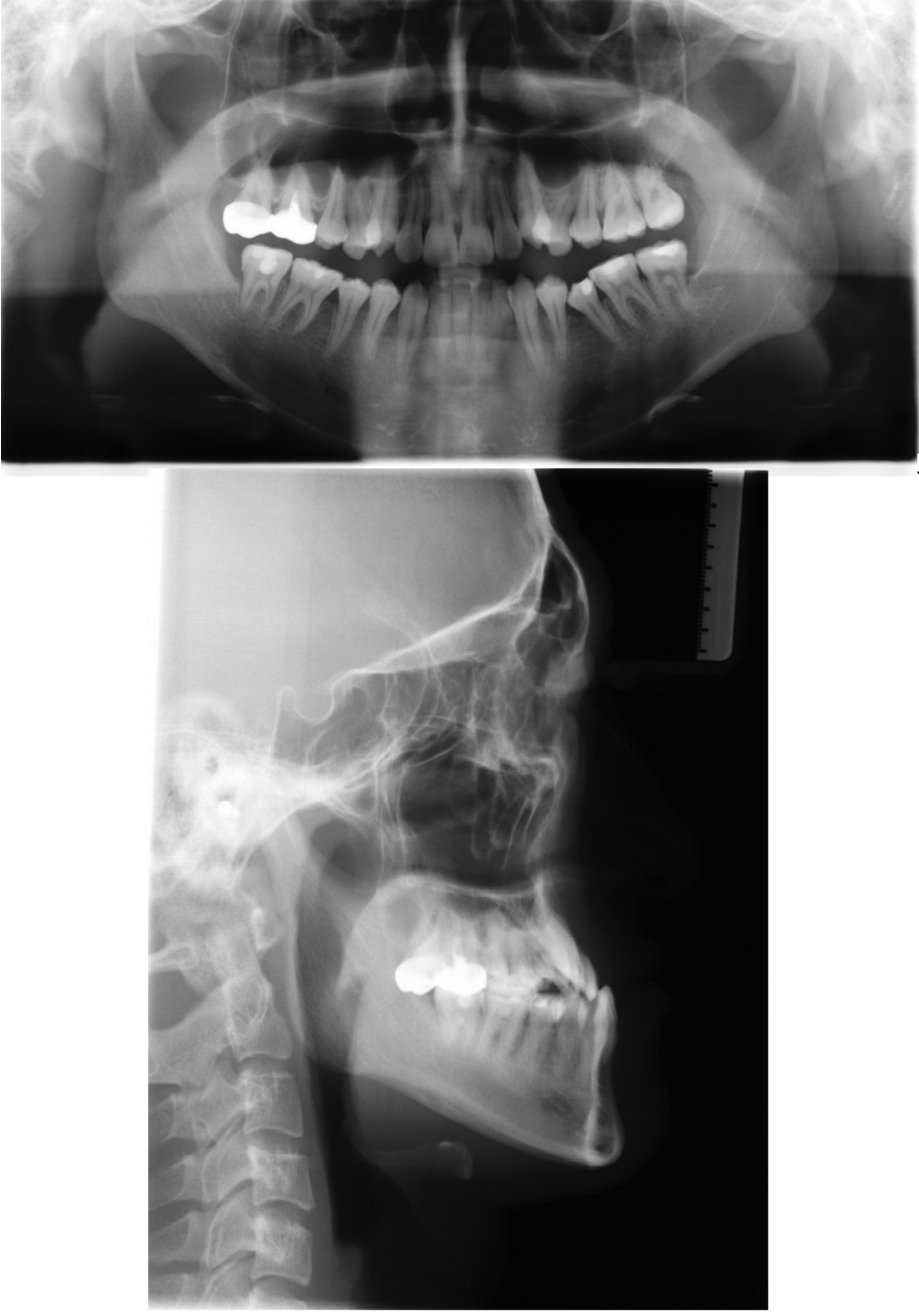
Pretreatment panoramic radiograph and lateral cephalogram.
